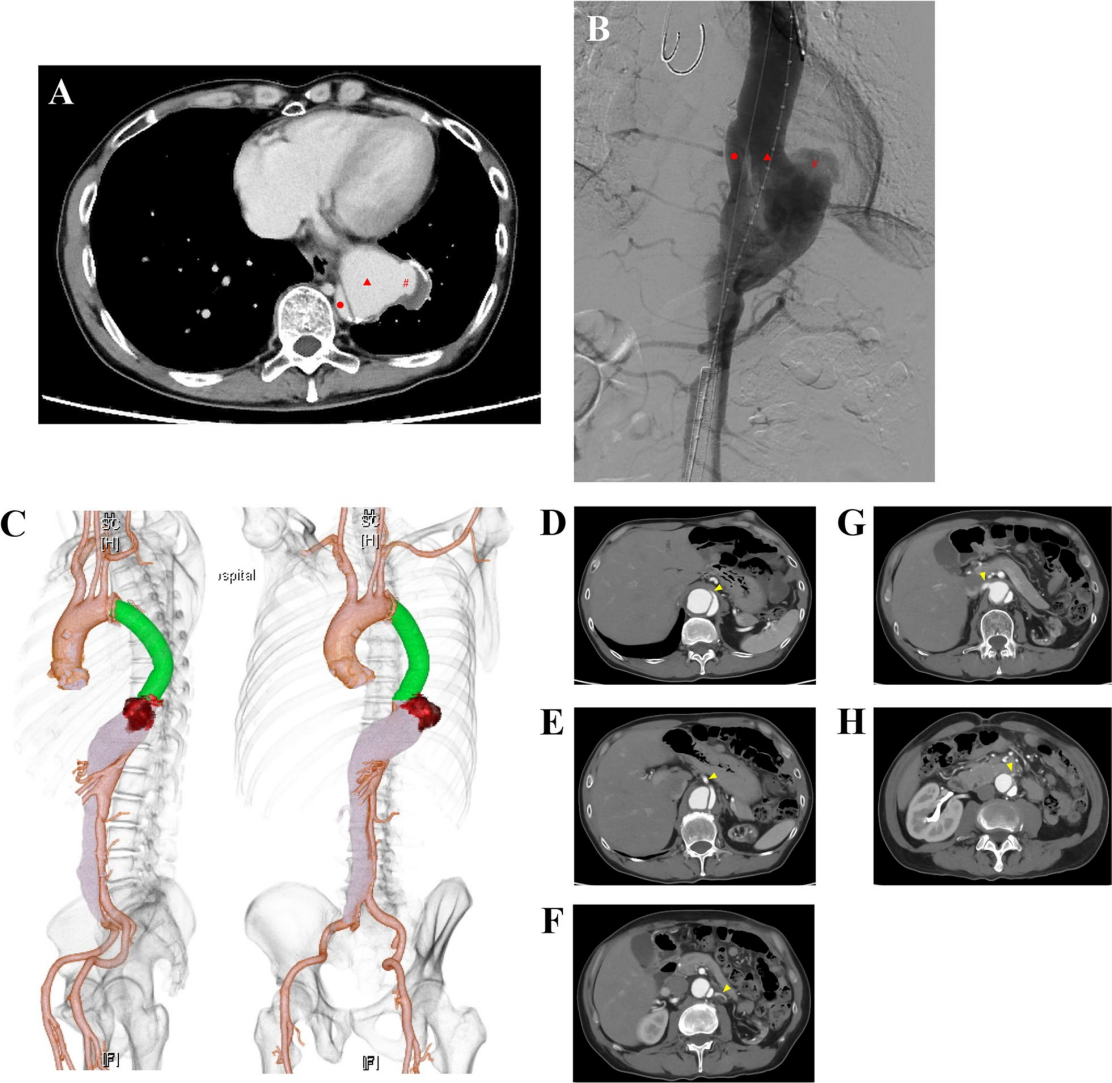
Preoperative findings. A Contrast-enhanced computed tomography (CT) scan. B Angiography of an expanded aneurysm with the saccular aneurysm. Each marker (●, ▲, and #) shows the same. C Three-dimensional-CT. D–H Celiac artery (D), superior mesenteric artery (E), and left renal artery F from the true lumen. Right renal artery (G) and inferior mesenteric artery H from the false lumen. Each artery is shown as a yellow triangle
